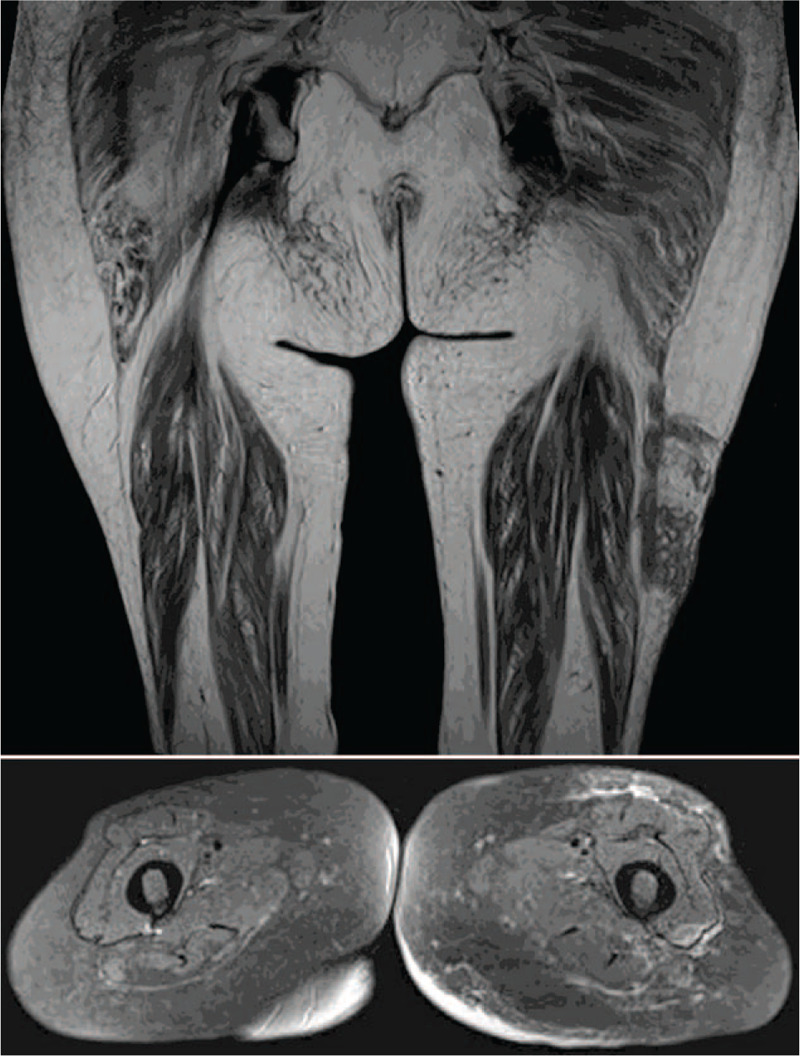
Case 1. MRI showed a lobular margined 15 cm mass in the intermuscular septum between left vastus lateralis and biceps muscles.
